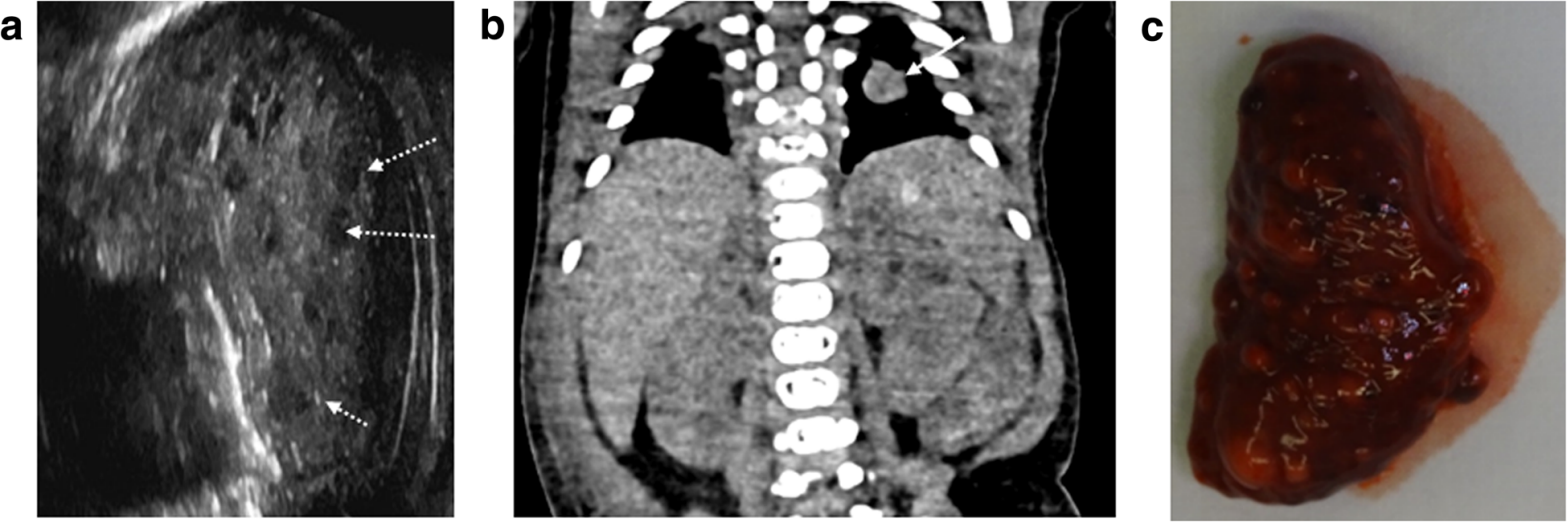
a–c Multiple splenic metastases from infantile fibrosarcoma in a 14 day old neonate (same case as in Fig. 9). Post-mortem imaging was performed 3 days after death. The post-mortem ultrasound in coronal section (a) demonstrates numerous hypoechoic lesions throughout the spleen (dotted arrows). On the corresponding coronal unenhanced CT image of the upper abdomen (b), the splenic lesions are less apparent, although a pulmonary metastasis in the left lung (solid arrow) is noted. At autopsy, the extracted spleen (c) reveals numerous solid lesions
